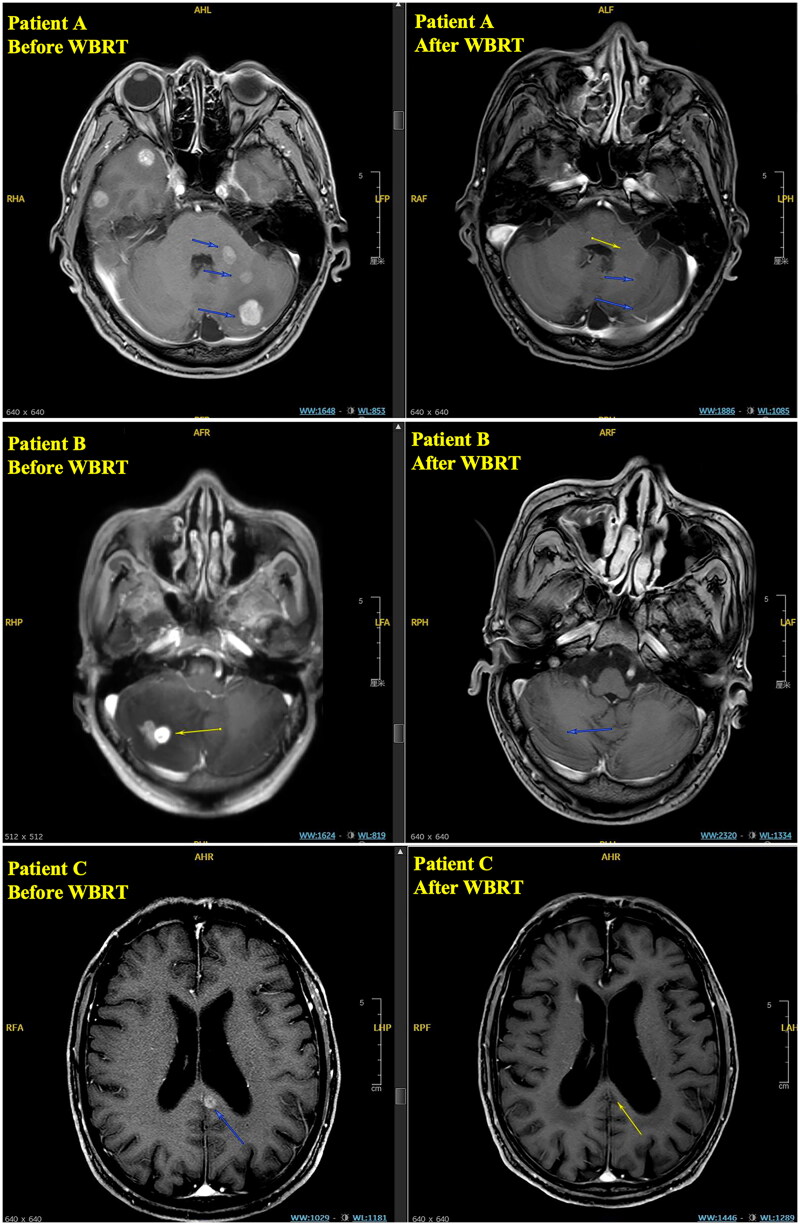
The enhanced T1-weighted MRIs of three representative patients who achieved CR response in intracranial lesions.
